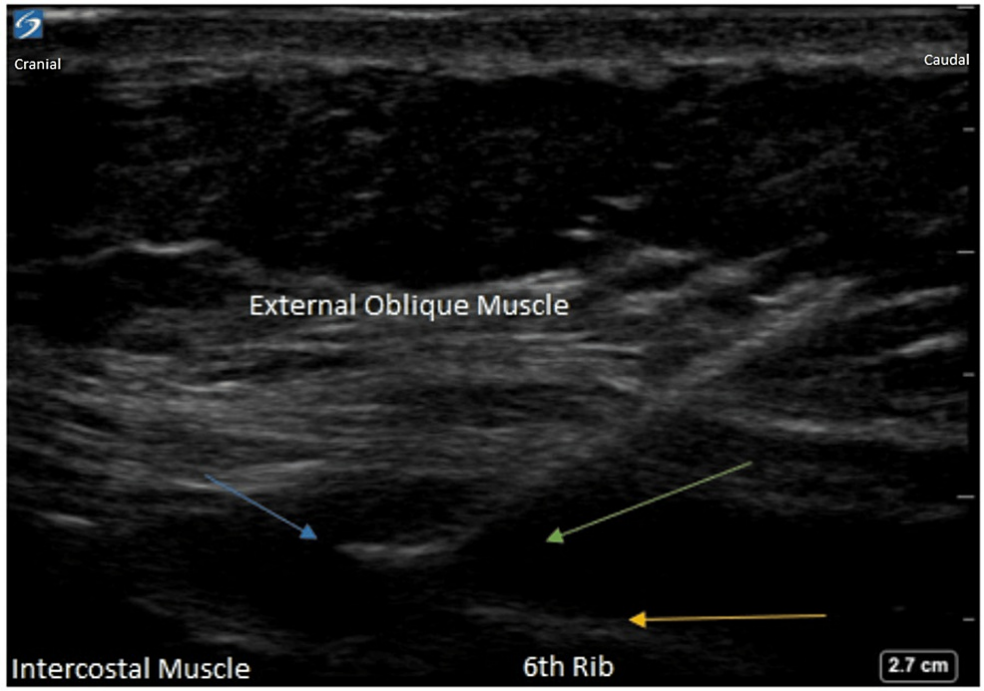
Ultrasound-guided external oblique intercostal block. Green arrow: injected solution; blue arrow: tip of the needle; yellow arrow: sixth rib.
